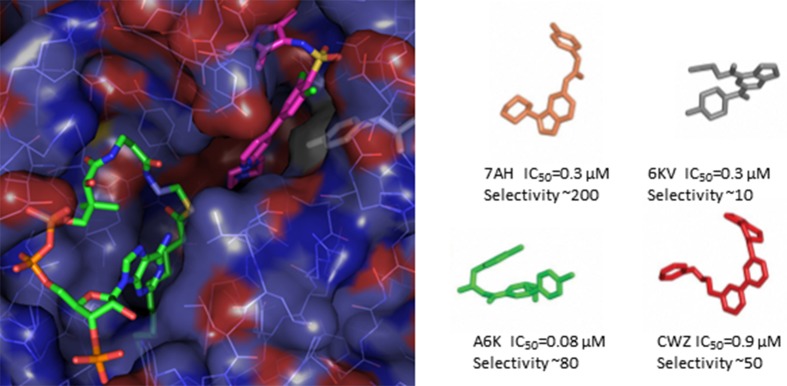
Left: the X-ray structure of LmNMT (2wsa) in complex with DDD646 (magenta C atoms) occupying the substrate binding site. The adjacent active site is occupied by myristoyl-CoA (green C atoms). Tyr217 is highlighted in white. Right: the four ligands complexed with LmNMT described in the current paper and also found to occupy the substrate binding pocket.
